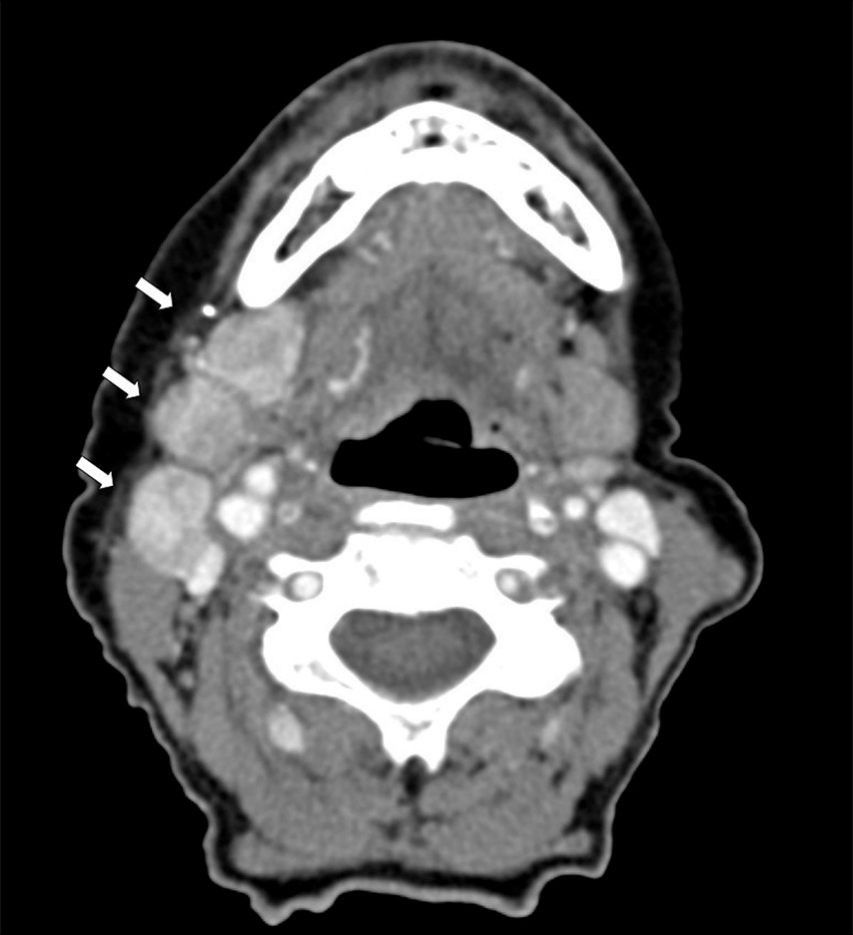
Axial CT with contrast demonstrating abnormal, hyperenhancing right levels 1B and 2 lymphadenopathy (white arrows).
